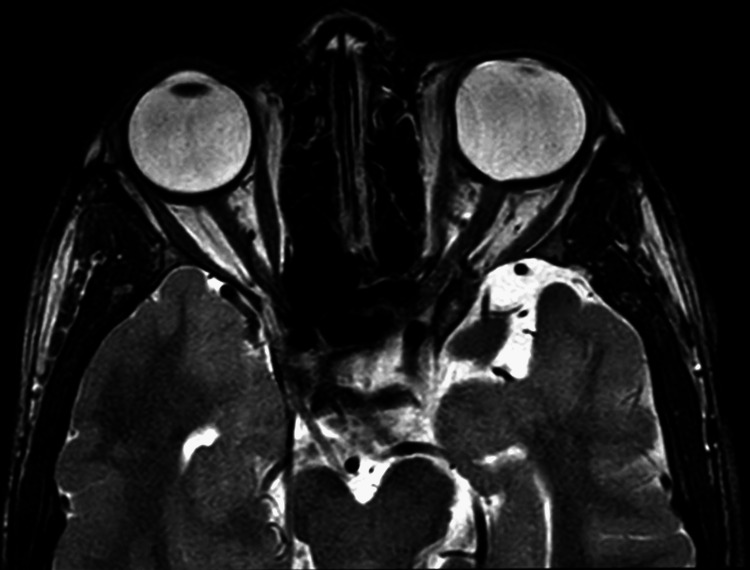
MRI-axial section T2WI of bilateral orbit showing a mild thickening of the optic nerves
